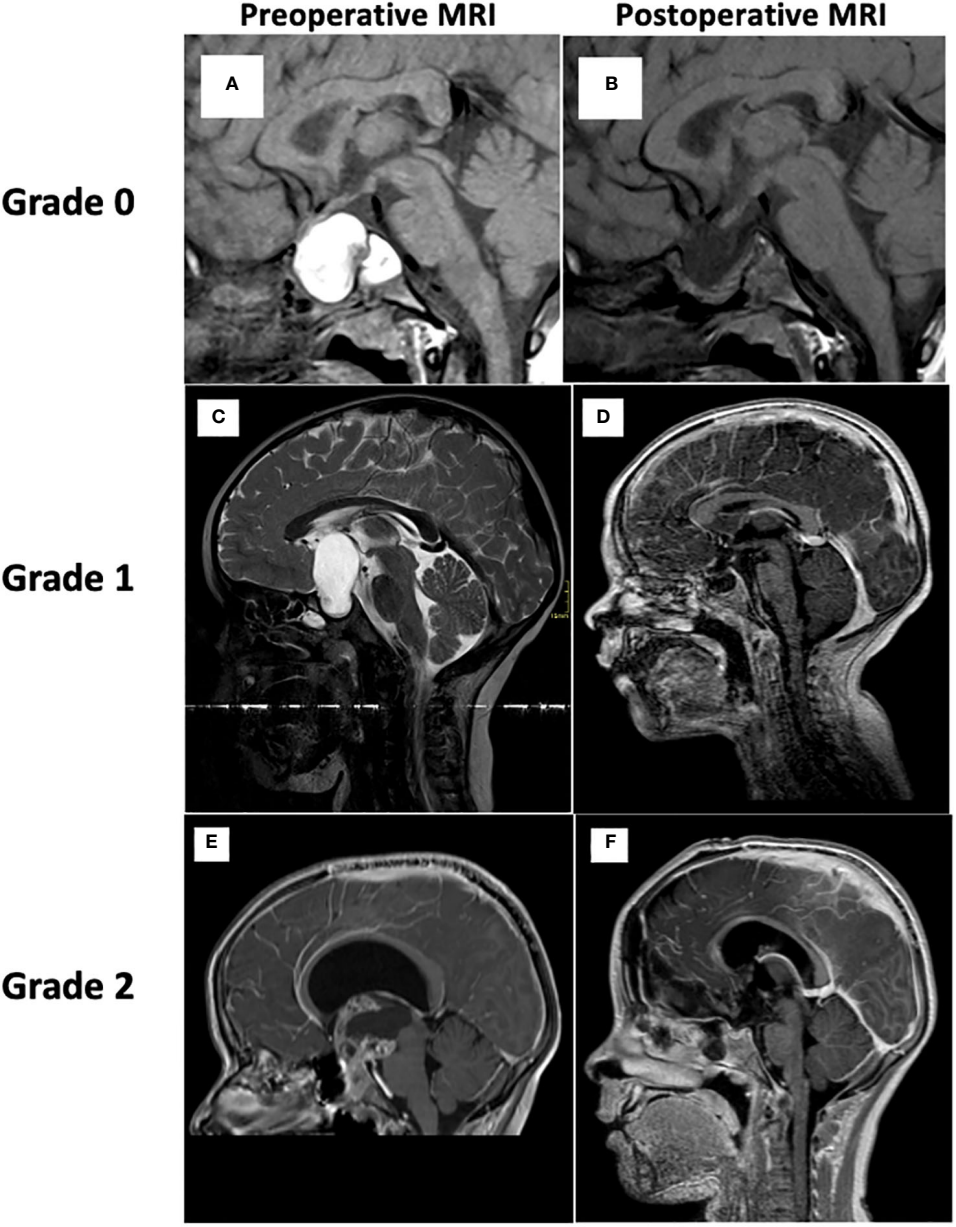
Brain MRI and grading according to Müller’s classification. Grade 0. Reference from: Otte A, Müller HL. Childhood-onset Craniopharyngioma. J Clin Endocrinol Metab. 27 sept 2021;106(10):e3820−36. (A) Preoperative sagittal T1-WI shows a predominantly cystic lesion in the sella turcica and the suprasellar cistern. No mass effect is visible on the infundibulum, the floor of the third ventricle or the mamillary bodies. (B) Postoperative sagittal T1-WI. Grade 1. (C) Preoperative sagittal T2-WI shows a predominantly cystic mass of the hypothalamic-pituitary region pushing back the floor of the third ventricle (no mass effect on the mamillary bodies). (D) Postoperative sagittal T1-WI with Gadolinium shows a complete resection of the tumor (no tumor residue). Grade 2. (E) Preoperative sagittal T1-WI after contrast administration, shows a solid and cystic mass in the hypothalamo-pituitary region compressing and invading the anterior and posterior hypothalamus. (F) Postoperative sagittal T1-WI after gadolinium, shows mass effect resolution. *MRI, magnetic resonance imaging; WI, weighted imaging*.
